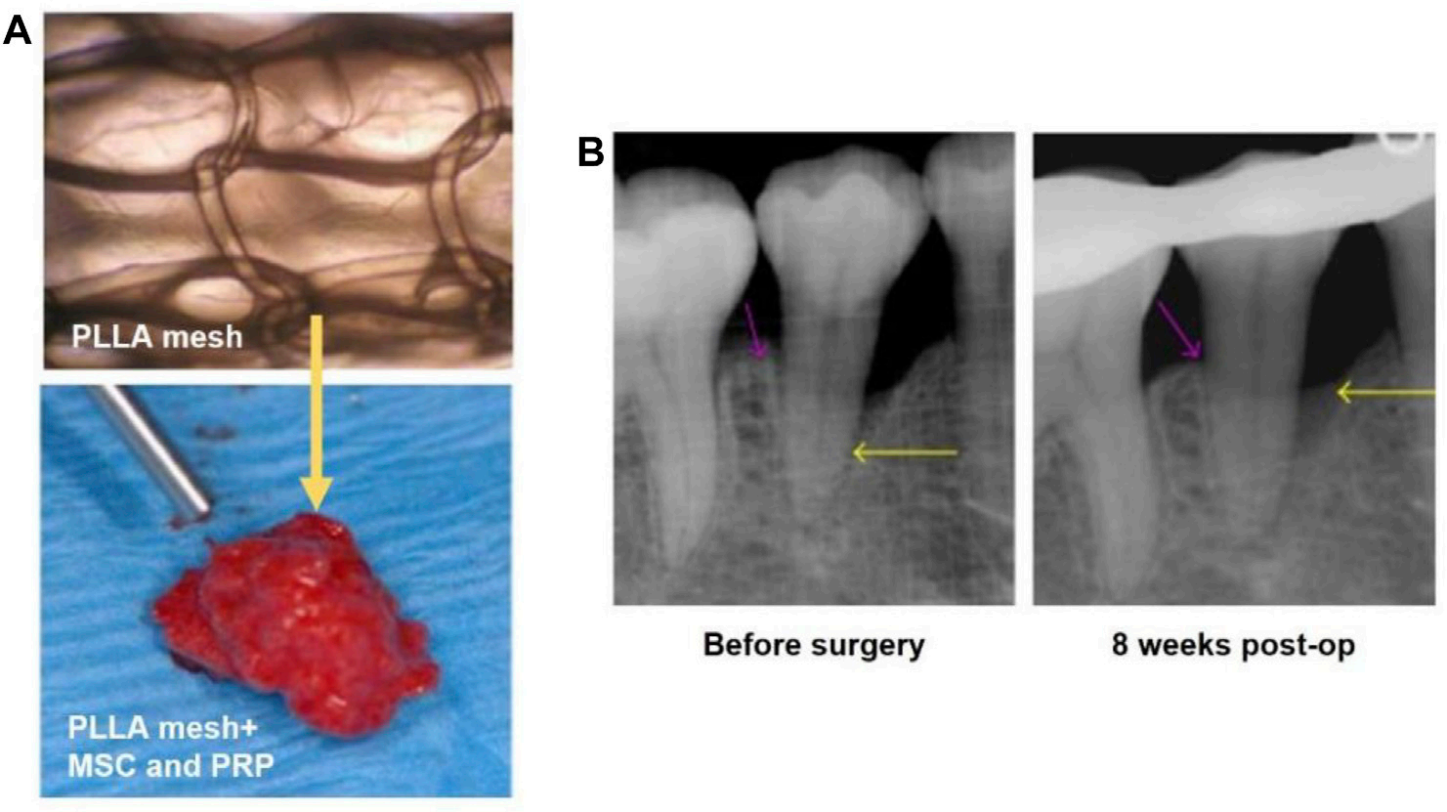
(A) The basket-shaped PLA mesh (top). The BMSCs and PRP were perfused in the PLA mesh for cell delivery (bottom). Reproduced with permission (Baba et al., 2016). Copyright 2016, Shunsuke Baba et al. (B) X-ray images showing the depth of the intrabony defects before and at 8 weeks post surgery as indicated by the yellow arrows. Reproduced with permission (Baba et al., 2016). Copyright 2016, Shunsuke Baba et al.
